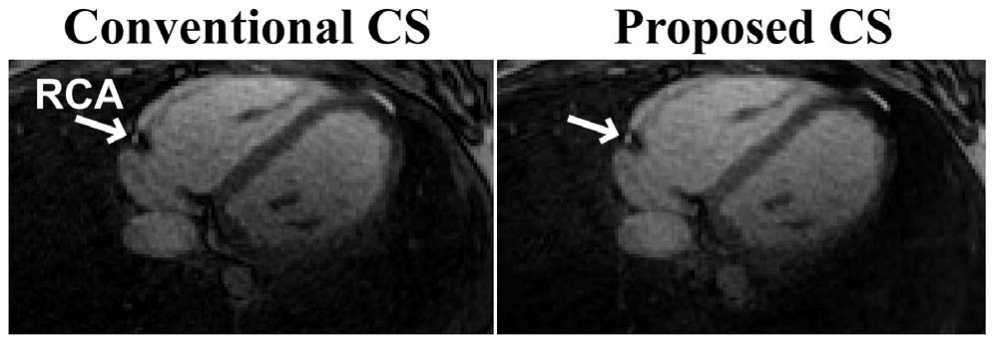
An example axial slice from a 3D radial whole heart MRI dataset at 20% sampling density, reconstructed with conventional CS reconstruction (left), and the proposed CS reconstruction (right), both with wavelet domain regularization. A cross section of the right coronary artery (RCA) is visualized clearly with both techniques.
